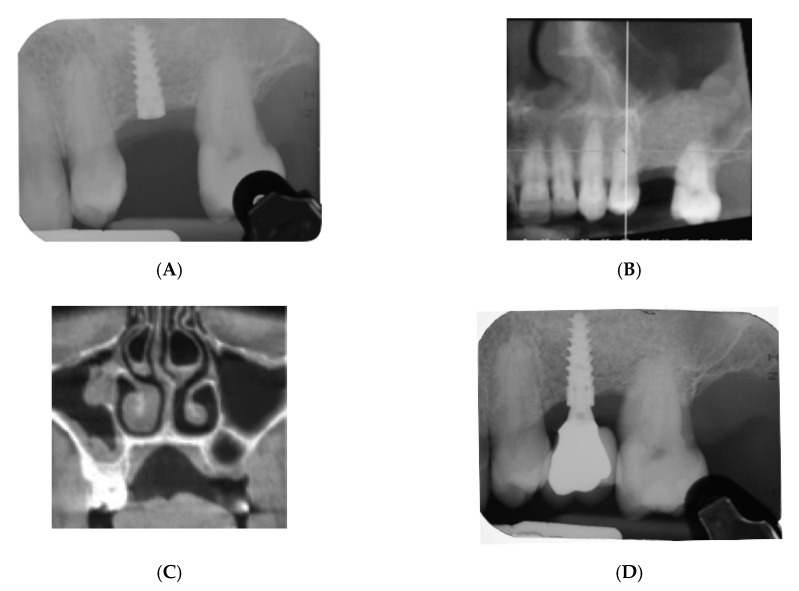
Radiographic images of a case with a residual bone height of 3.5 mm: (A) periapical radiograph showing posterior residual bone; (B) image taken soon after the procedure of lateral sinus lift; (C) implant positioned after 5 months; (D) 1-year follow-up after loading.
